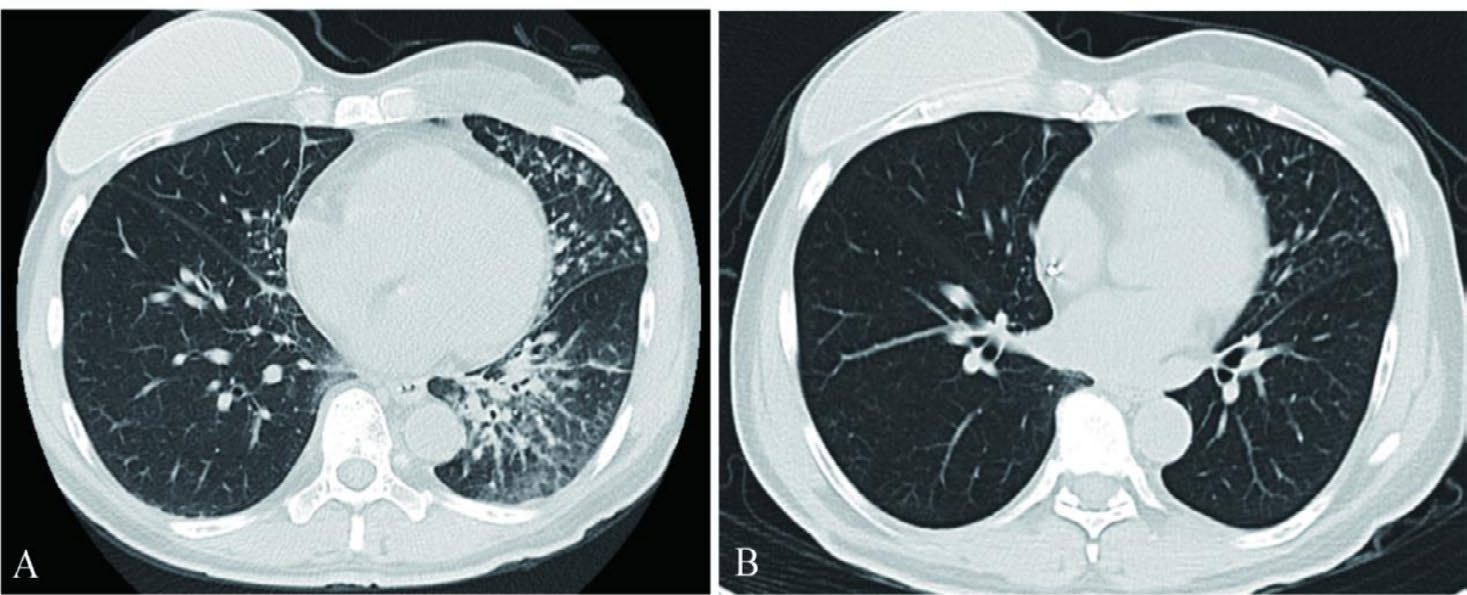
(A) Chest HRCT (recurrent CT findings in the left lung–interstitial pneumonia). (B) Chest HRCT (after 3 cycles of T‐DXd treatment).
